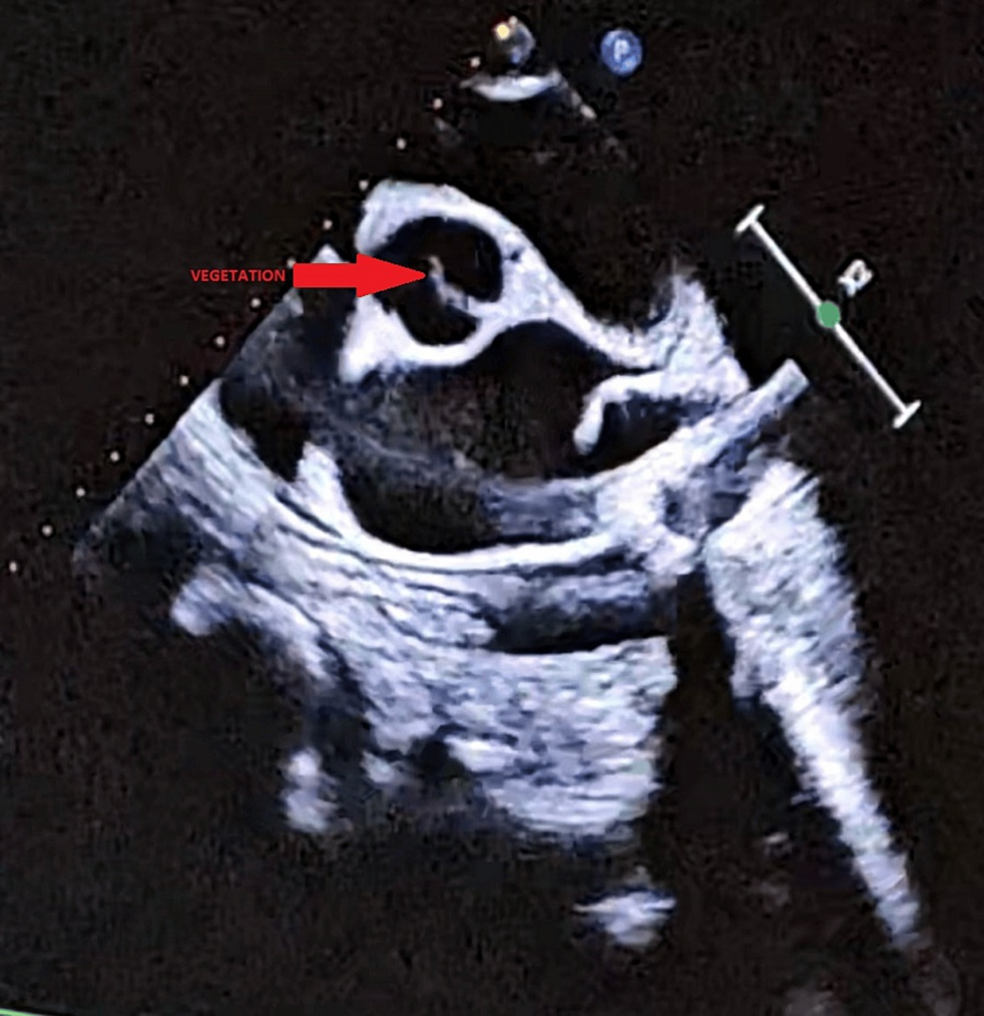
A 2D echocardiogram showing vegetation on the aortic valve leaflet. 2D, two-dimensional
